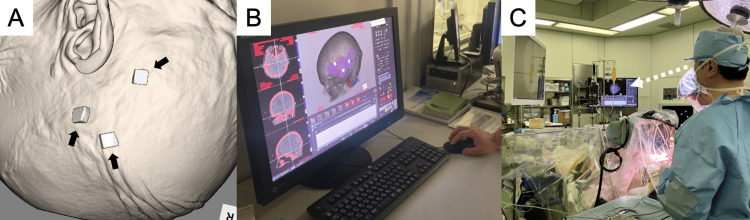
The utilization of 3D images for preoperative planning and intraoperative reference. The attachment of three or four CT-visible markers to the patient's head skin facilitates accurate localization of the hematoma. We referred to this technique as "Marking CT" (A). The 3D images are created using Ziostation2 software (Ziosoft Inc., Tokyo, Japan), performed by trained radiographers (B). Surgeons utilize these 3D images for intraoperative reference and to maintain orientation during endoscopic hematoma evacuation (C).
